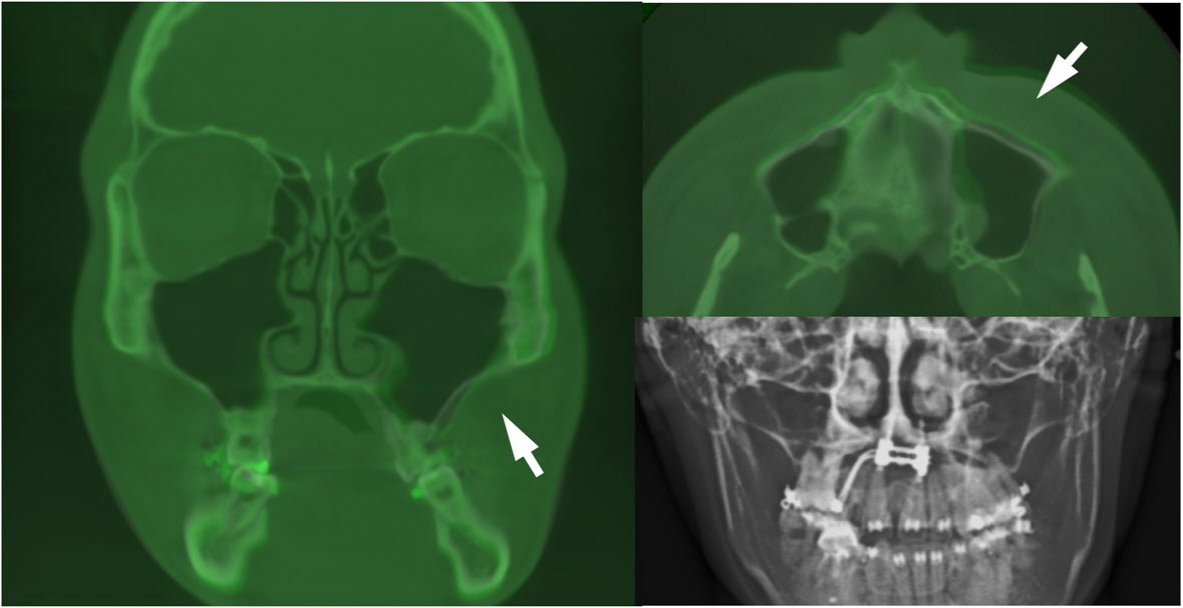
This is another case with an unfavorable expansion result using the traditional MSE fabrication process: unusual expansion pattern (white arrow) revealed by CBCT superimposition (white line, initial; green line, post-expansion). The canted palatal plane caused the right TADs inclination toward the maxillary suture during inserting MSE. The appliance in this case was thus removed to allow relapse of the dentoalveolar tissue
